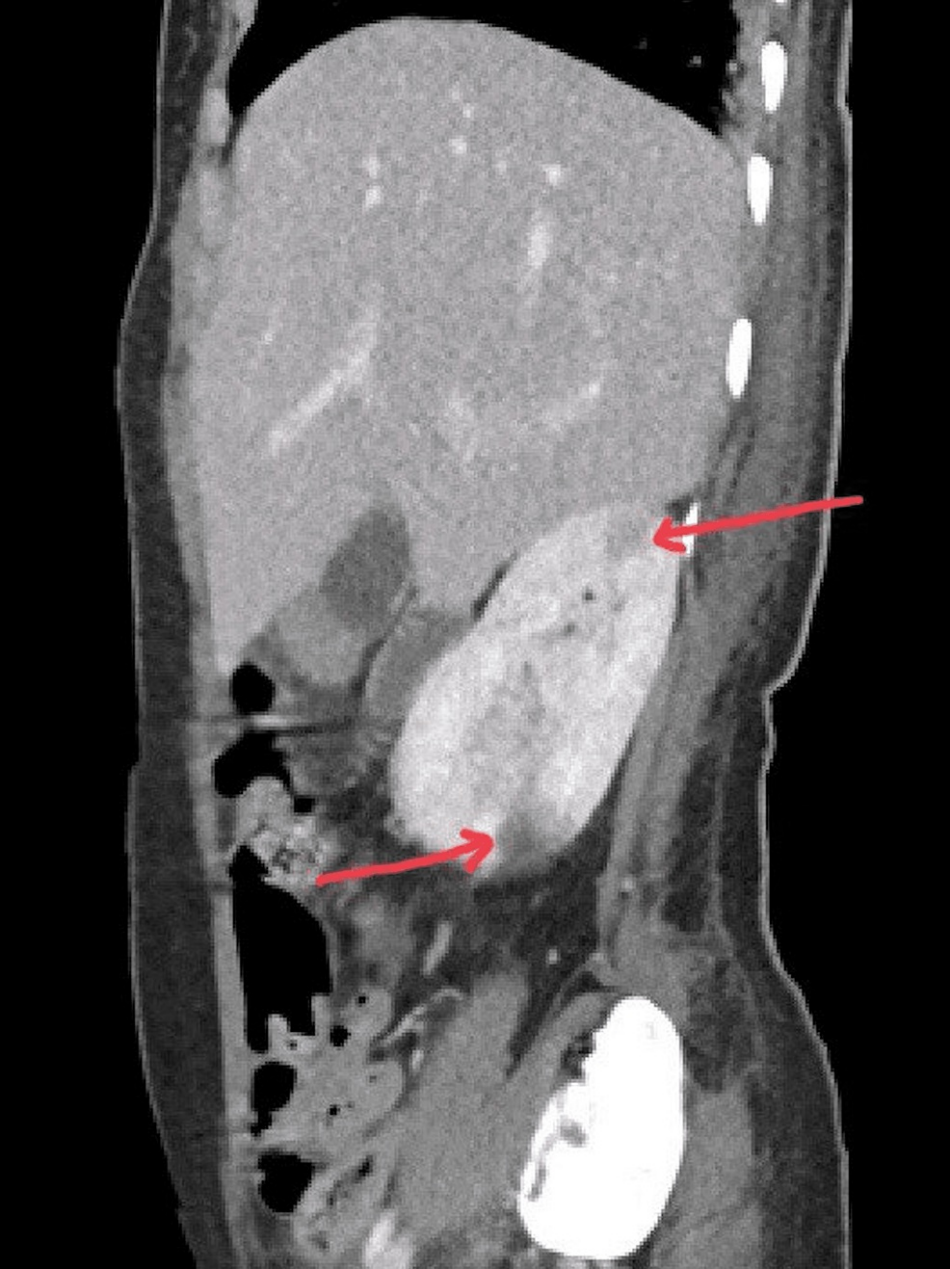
Sagittal view of CT abdomen shows multiple hypodense lesions likely representing infarcts.
